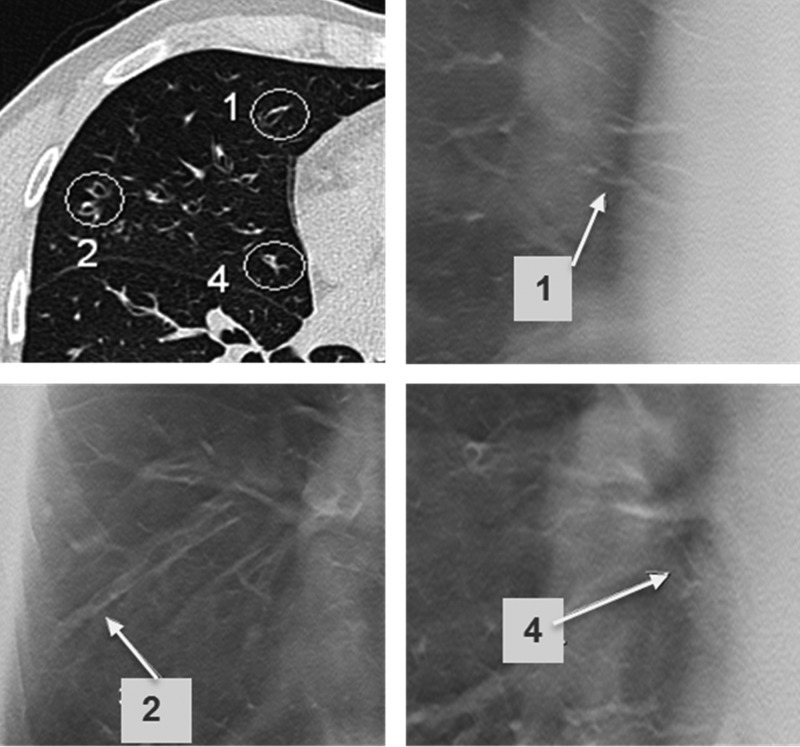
Examples of peripherally and centrally located dilated bronchi in the right middle lobe. Structure 1 is a peripheral bronchiectasis in the anterior region. Visibility score ranged from not visible (0, pulmonologist) to equal visibility (3, experienced radiologist). Structure 2 is a peripheral bronchiectasis with distal mucus plugging. All observers reported visibility superior to CT (Grade 4). Structure 4 is a central bronchus with thickened wall located close to the heart and great vessels. All observers assessed visibility as slightly inferior to CT (Grade 2).
